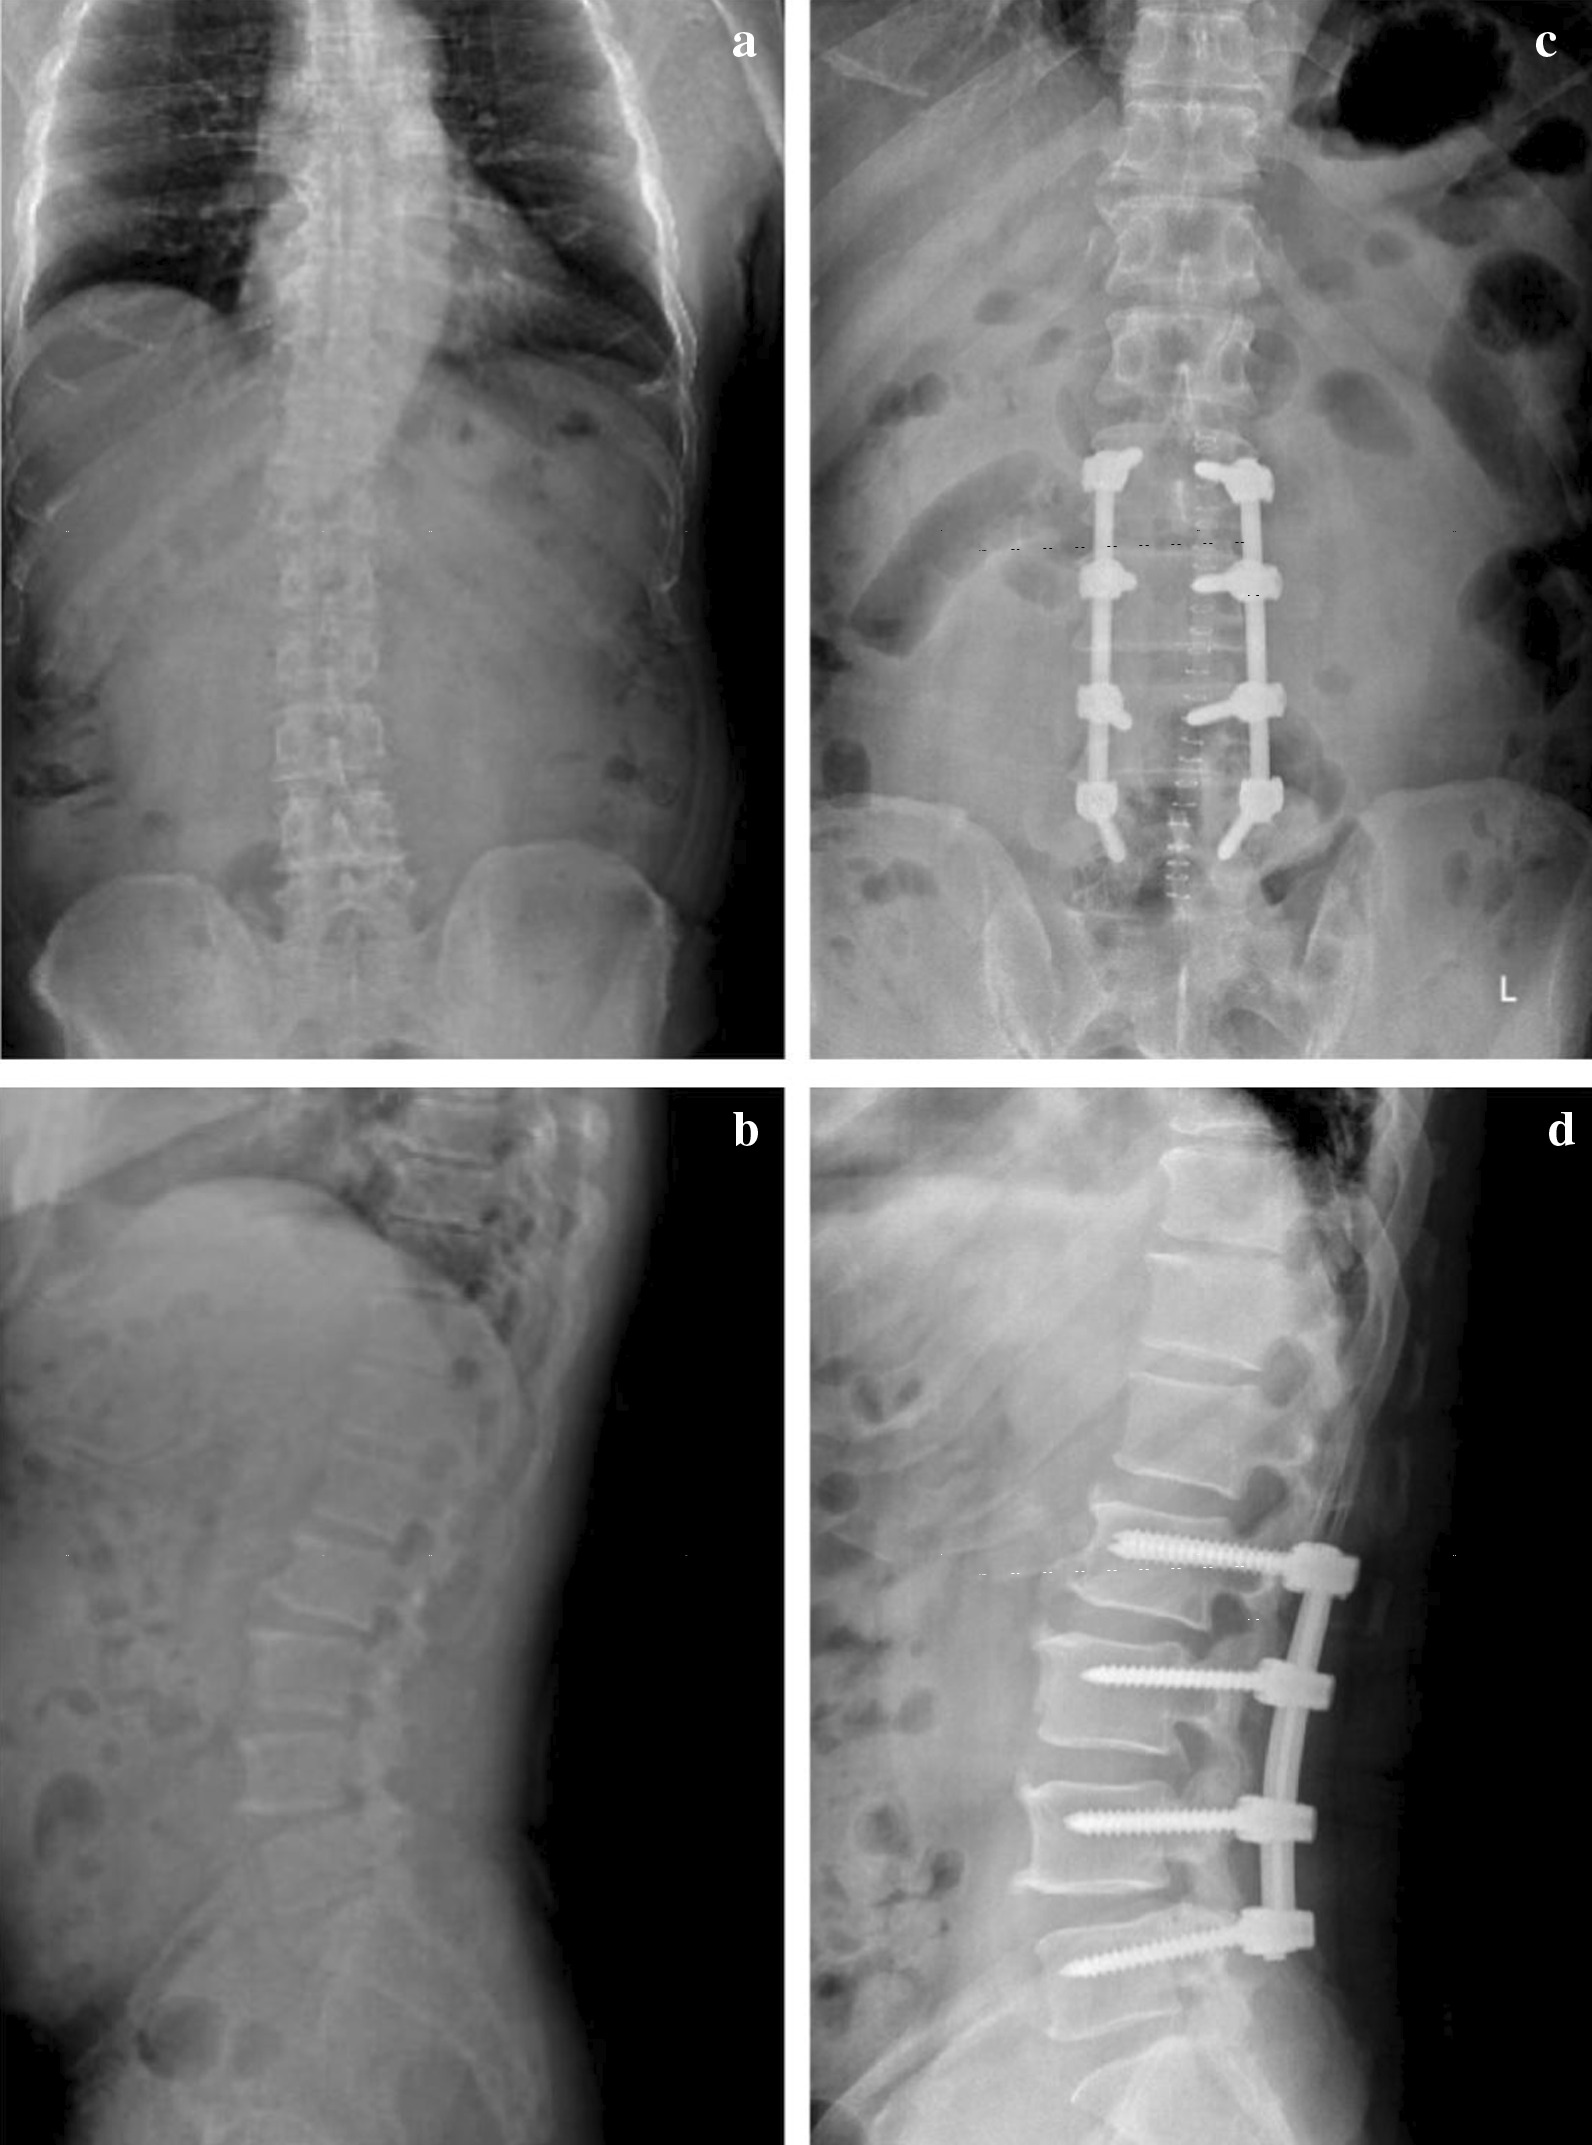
Preoperative and immediate postoperative X-Ray. Immediate postoperative X-Ray image in comparison of the preoperative counterparts shows satisfactory location of graft. a Preoperative coronal image. b Preoperative sagittal image. c Postoperative coronal image. d Postoperative sagittal image
